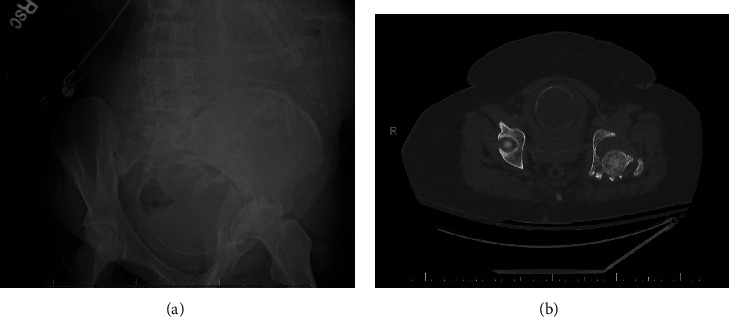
(a) AP radiograph and (b) axial CT scan of the pelvis obtained in the initial trauma evaluation, demonstrating a left transverse posterior wall acetabulum fracture with concurrent hip dislocation in this patient with a gravid uterus.
